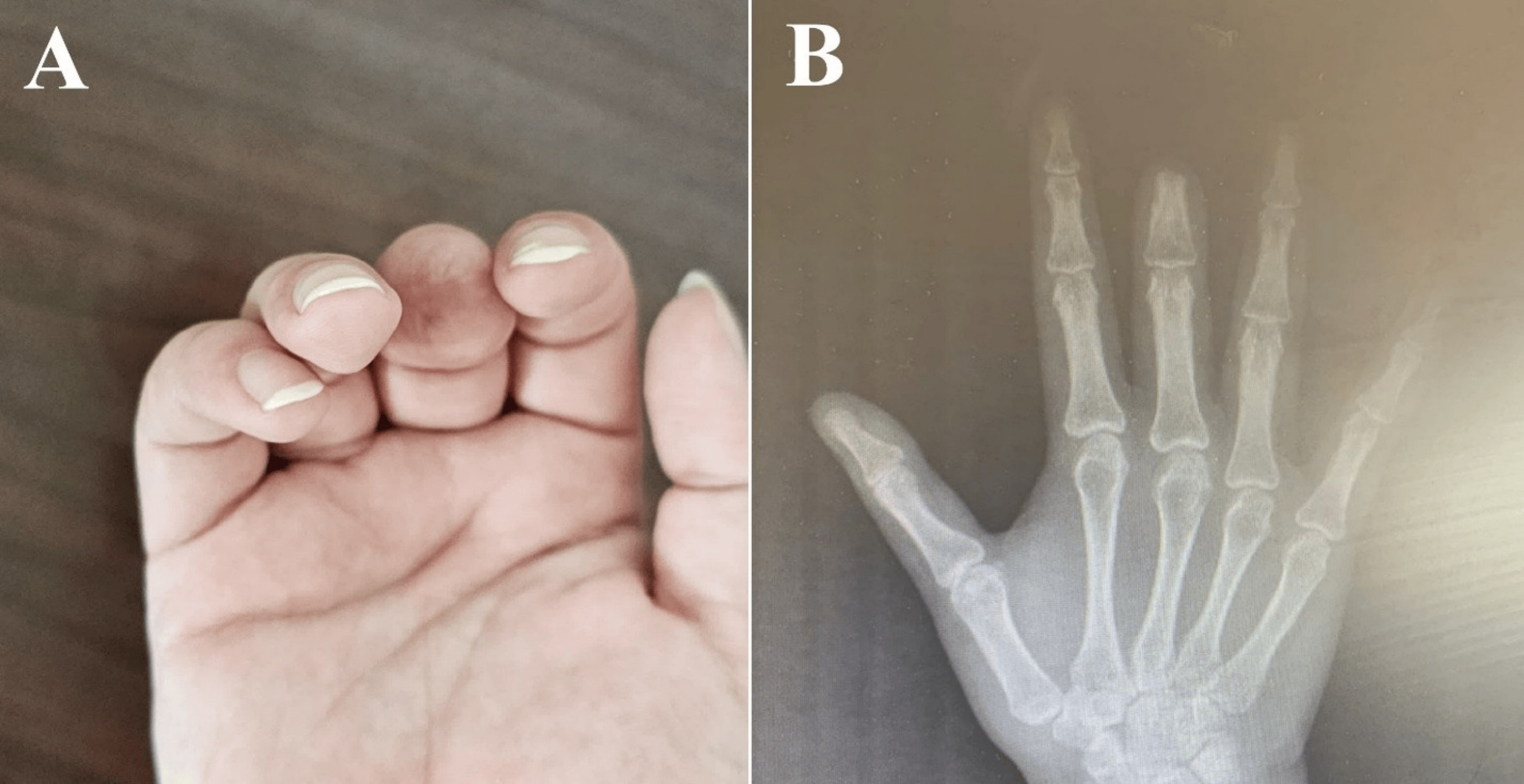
(A) Clinical image showing amputation at the level of the middle phalanx of the middle finger. (B) Radiographic image demonstrating amputation at the level of the head of the middle phalanx of the middle finger.
